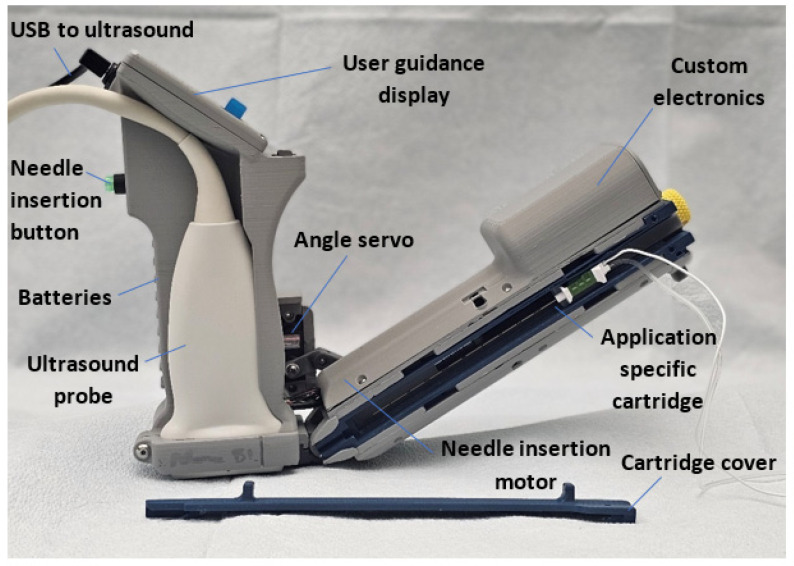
The full prototype AI-GUIDE-Nerve Block device includes two connected arms, including one arm that holds an ultrasound probe, user guidance display, and a needle insertion button, and the other holds a cartridge for a needle to be inserted with a motor.
